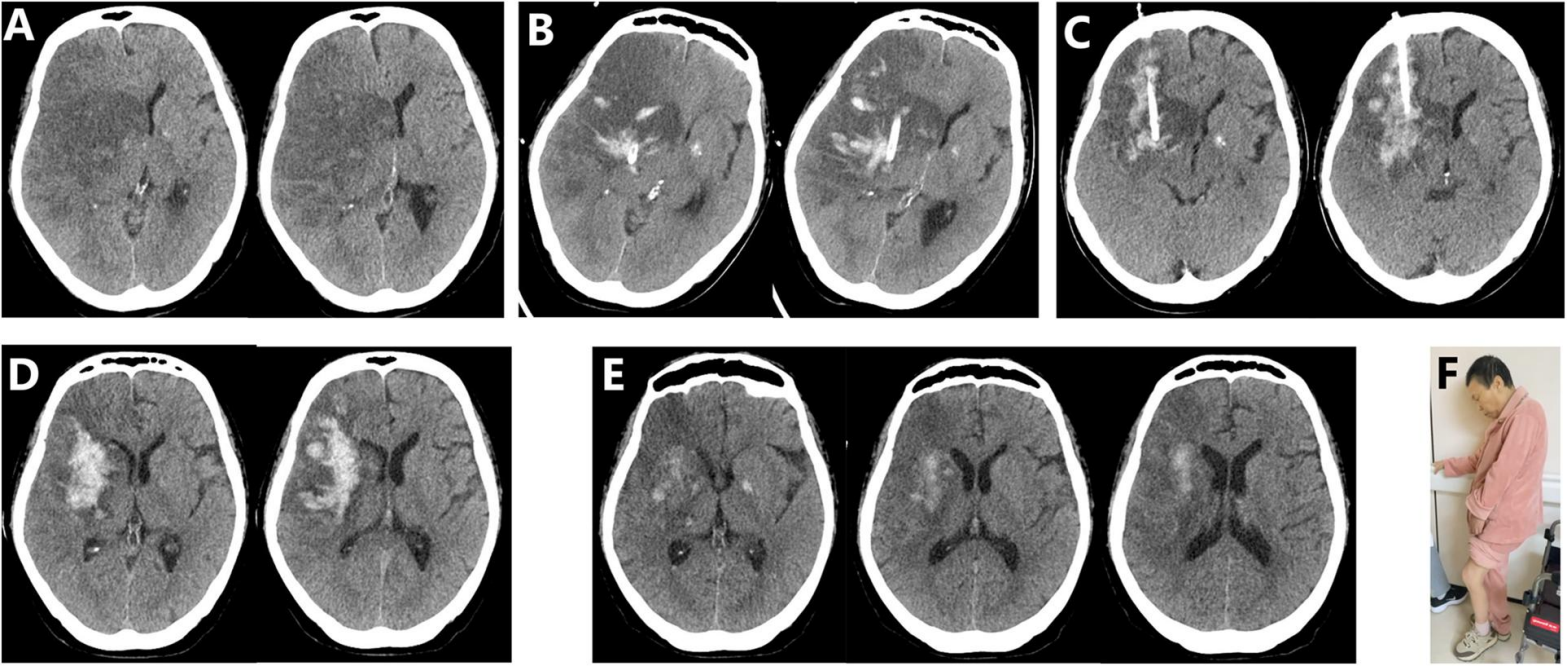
Surgical procedure and postoperative follow-up of a 70-year-old female patient with malignant middle cerebral artery infarction. (A) Preoperative computed tomography (CT) showed a 13 mm midline shift and a completely compressed lateral ventricle ipsilateral to the infarction. (B) CT scan on day 1 after the operation showed that the midline shift did not worsen, but the mass effect was obvious. Subsequently, necrotic brain tissue was aspirated again. (C) CT scan on day 3 after the operation showed that the mass effect was relieved. (D) CT scan on day 6 after the operation showed that the midline shift had almost returned to normal. There was a small amount of bleeding within the infarct area, but no mass effect was observed. (E) CT scan 2 weeks after the operation showed that the midline shift fully recovered and the hemorrhage in the infarct area was absorbed. (F) After 6 months of follow-up, the patient could walk independently using a cane and the mRS score was 3.
